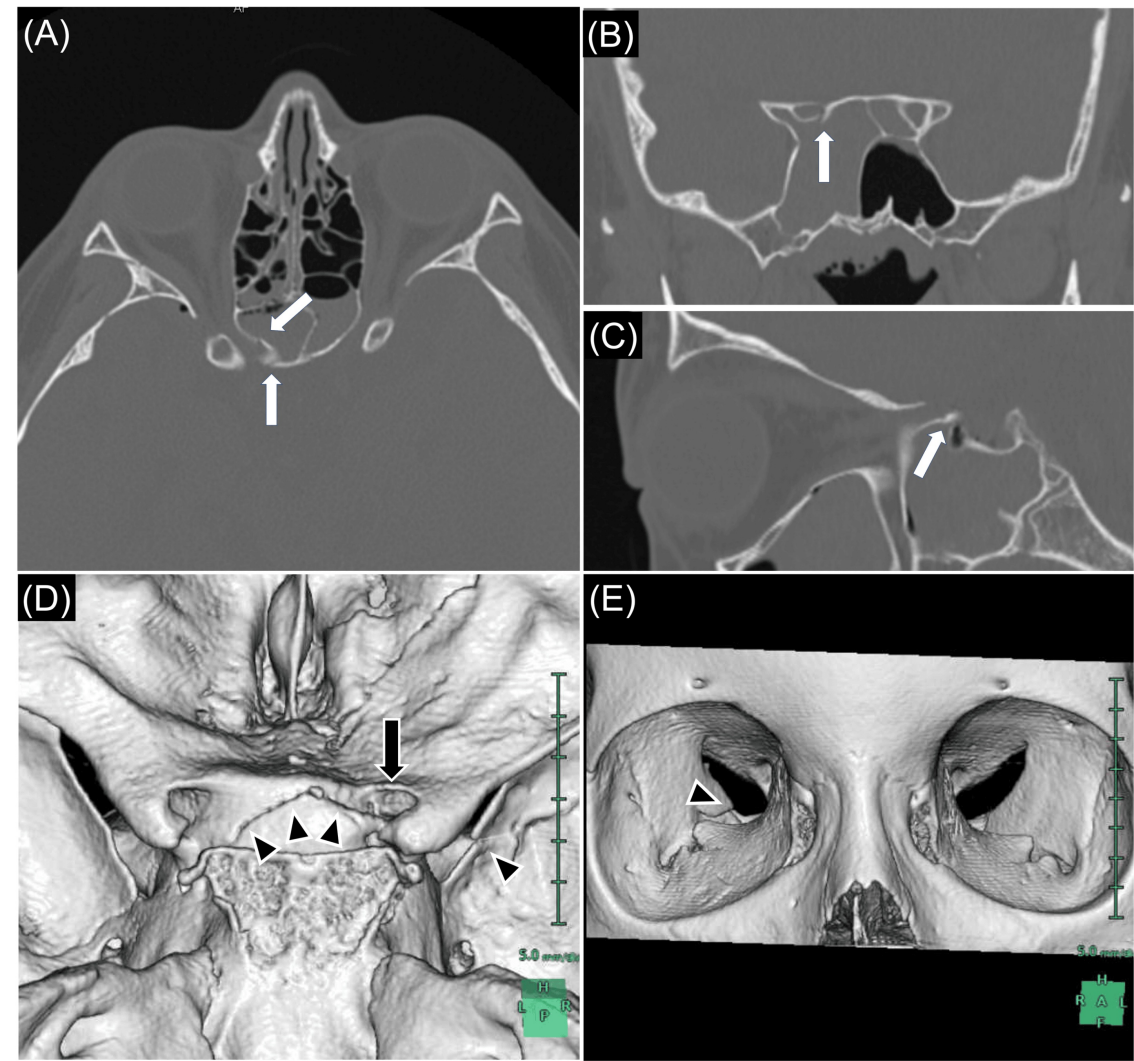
Bone window computed tomography (CT) at the initial examination (A): Axial view showing a right orbital wall fracture (white arrows) and pneumocephalus; (B): Coronal view showing that the fracture caused right optic canal narrowing (white arrow); (C): Sagittal view showing fractures at the right optic canal (white arrow) and pneumocephalus; (D) and (E): Three-dimensional CT (3D CT) revealing the fracture (black arrows) and the subsequent right optic canal narrowing (black arrowheads).
